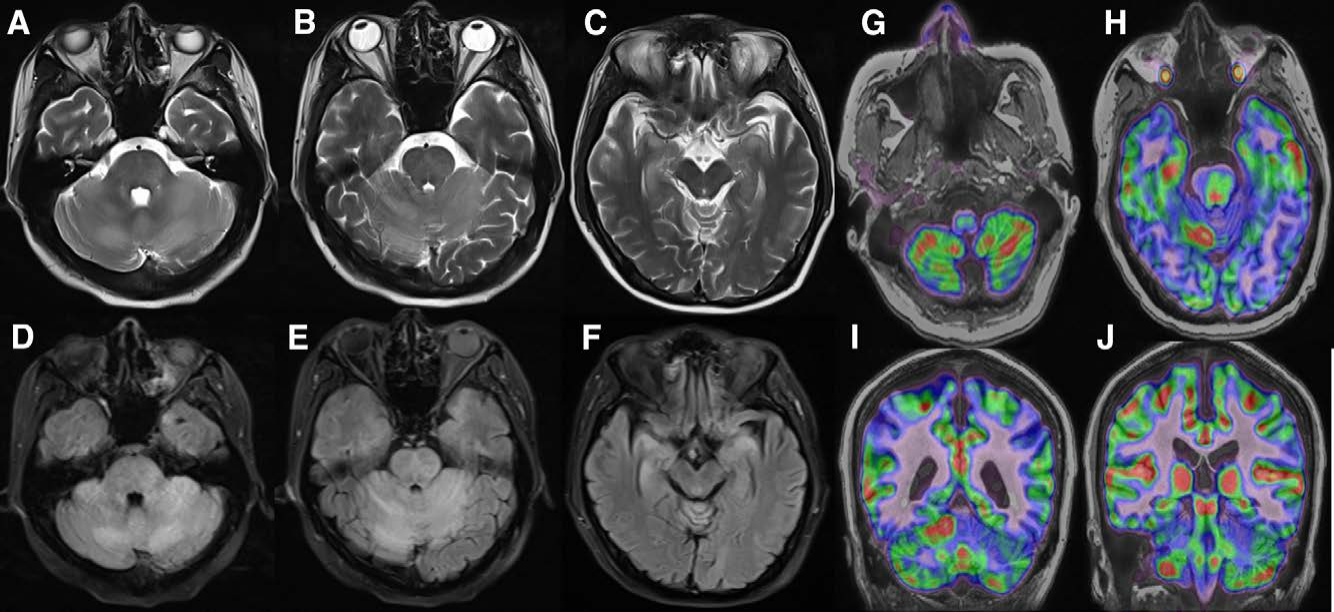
Representative images of brain lesion. (A–C) MRI T2WI, (D–F) MRI T2Flair: High signal in the right hippocampus, left insula, bilateral cerebellar hemispheres, and vermis, and the possibility of borderline encephalitis. (G–J) PET/CT fusion images: Intracranial abnormal signal with increased glucose metabolism, considered as autoimmune encephalitis. MRI = magnetic resonance imaging, PET = positron emission tomography, CT = computed tomography.
